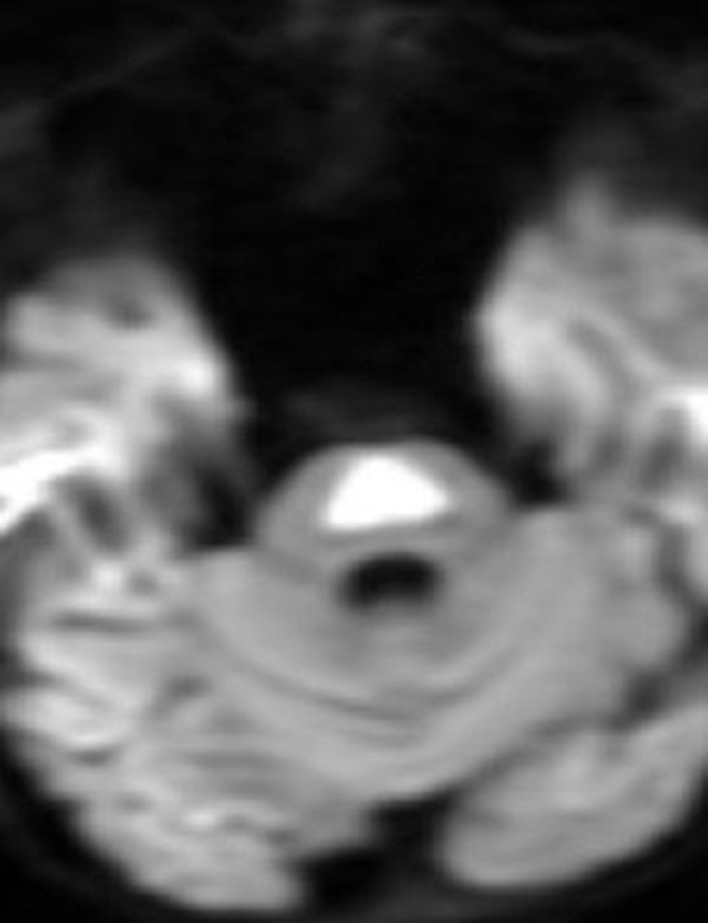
MRI brain, Axial DWI showing trident shaped diffusion restriction in the central portion of pons corresponding with fig-3
